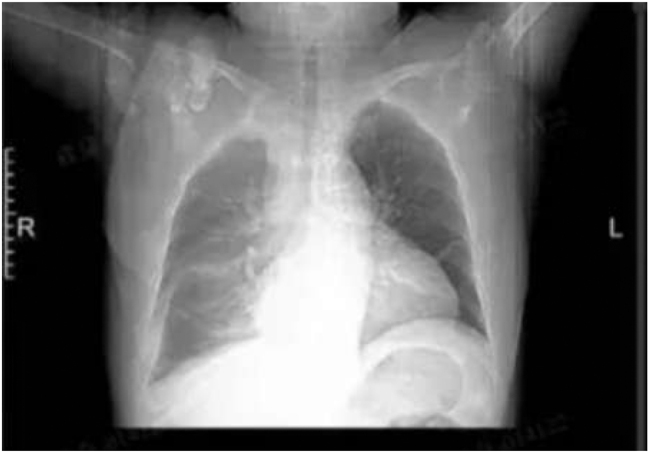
Chest CT showing pleural effusion and cardiomegaly.
